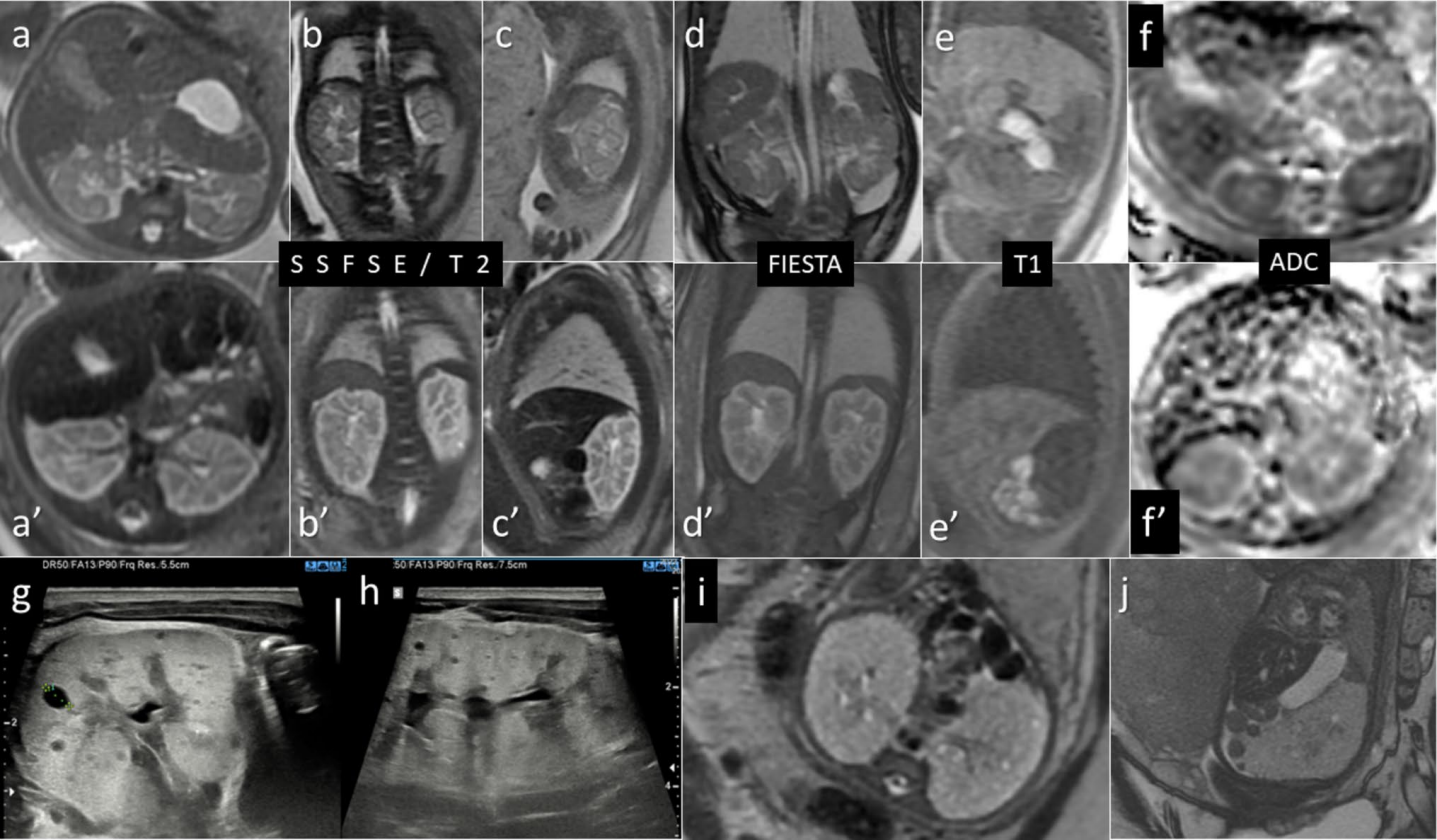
Comparison of the normal SI of the fetal kidneys (a–f, top row) and of the reversed pattern in case no. 1 (a'–f', middle row) in corresponding sequences and sections at the same gestational age of 36 weeks. Postnatal ultrasound of the cystic kidneys in neonate no. 1 at day of life 7 (g–h, bottom row). Completely different MRI appearance of ARPKD in a fetus for comparison (i–j, bottom row)
